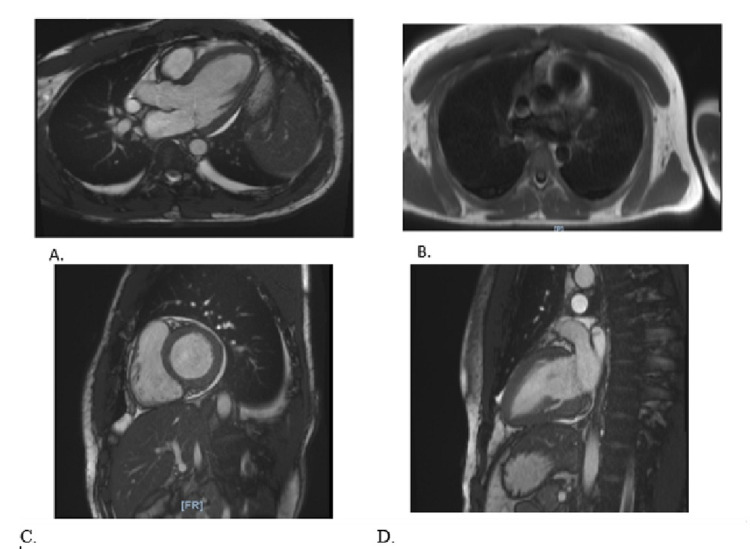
Cardiac MRI with gadolinium-enhanced contrast. Cardiac MRI is performed with bright blood and black blood technique pre- and post-contrast administration. Dynamic contrast enhancement and delayed myocardial viability/scarring images are obtained (A-D). There is myocardial edema at the mid-lateral wall to the apex (C and D). There is global hypokinesia on the cine views is trace bilateral pleural effusion and pericardial effusion (A and B). Aortic valve is tricuspid and without elevation of peak systolic velocity or abnormality of area grossly evident. End-diastolic volume is below normal and the end-systolic volume is above normal. All of the parameters are within normal. Right ventricular ejection fraction 42% and stroke volume almost identical to the left.
